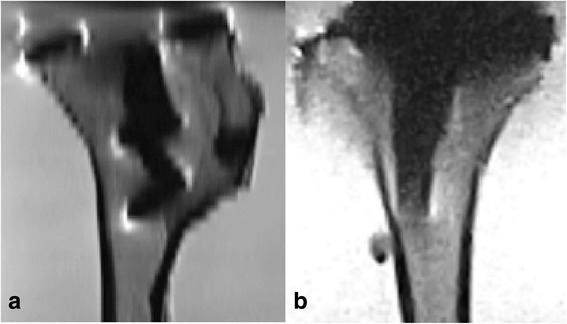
a MRI slices of the tibial component of a total knee prosthesis made with high field MRI with a TSE PD sequence in the sagittal direction. b MRI slices of the tibial component of a total knee prosthesis made with low-field MRI made with a TSE/FSE PD sequence in the sagittal direction
